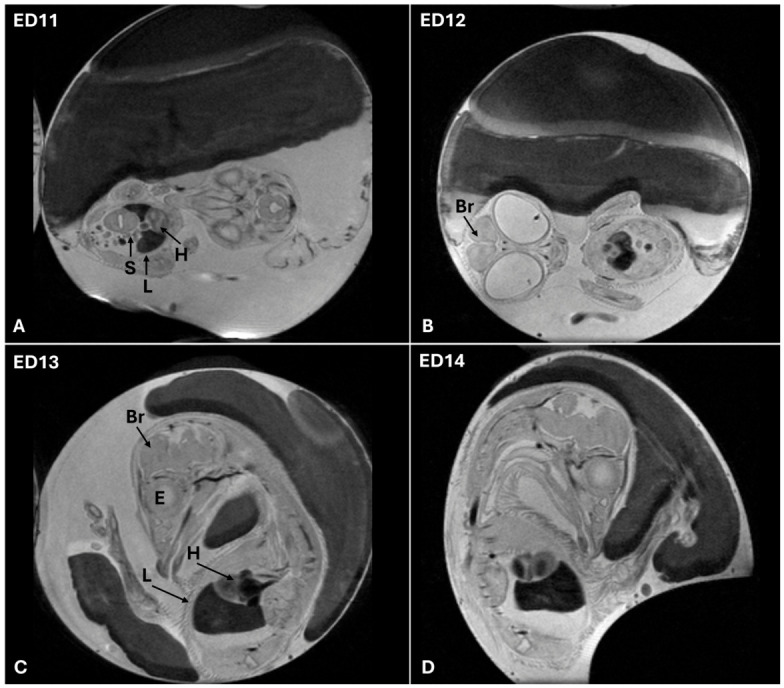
(A–D) T2-weighted MRI representative images of CE at ED11–ED14 showing advanced morphological maturation. Images were acquired in sagittal and paracoronal planes. Abbreviations: H, heart; L, liver; S, stomach; Br, brain; E, eye.
